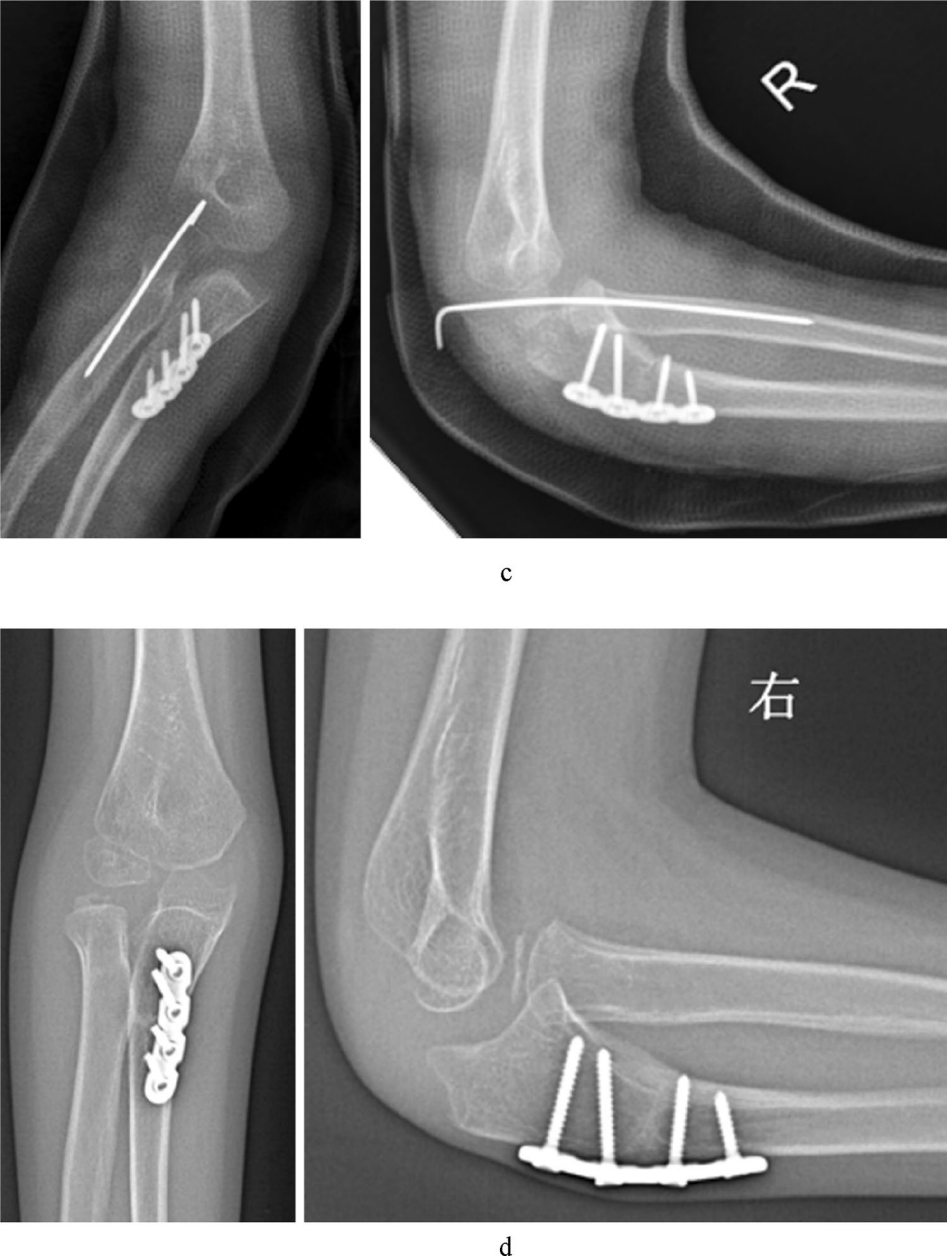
Imaging of a 7 years old boy (case 2) referred for the treatment of missed Monteggia lesion. His internal time between injury and operation was 8 months. (a) Preoperative X-ray. (b) Preoperative MRI. Axial T2-weighted images demonstrated the proximal radioulnar joint was dislocated and the appearance of the radial notch (the arrow) was flat; coronal T1- and T2-weighted images showed the radial notch of the ulna was hyperplasia (the arrow); sagittal T1-weighted images showed the dislocation of the humeroradial joint and the concave radial head (the arrow) combined with the folded and tortuousness annual ligament in the joint. (c) Open reduction and ulnar osteotomy with reconstruction of the annular ligament and a humeroradial pin was undertaken. (d) The radial head subluxated (Grade 3) at 2 years after operation.
